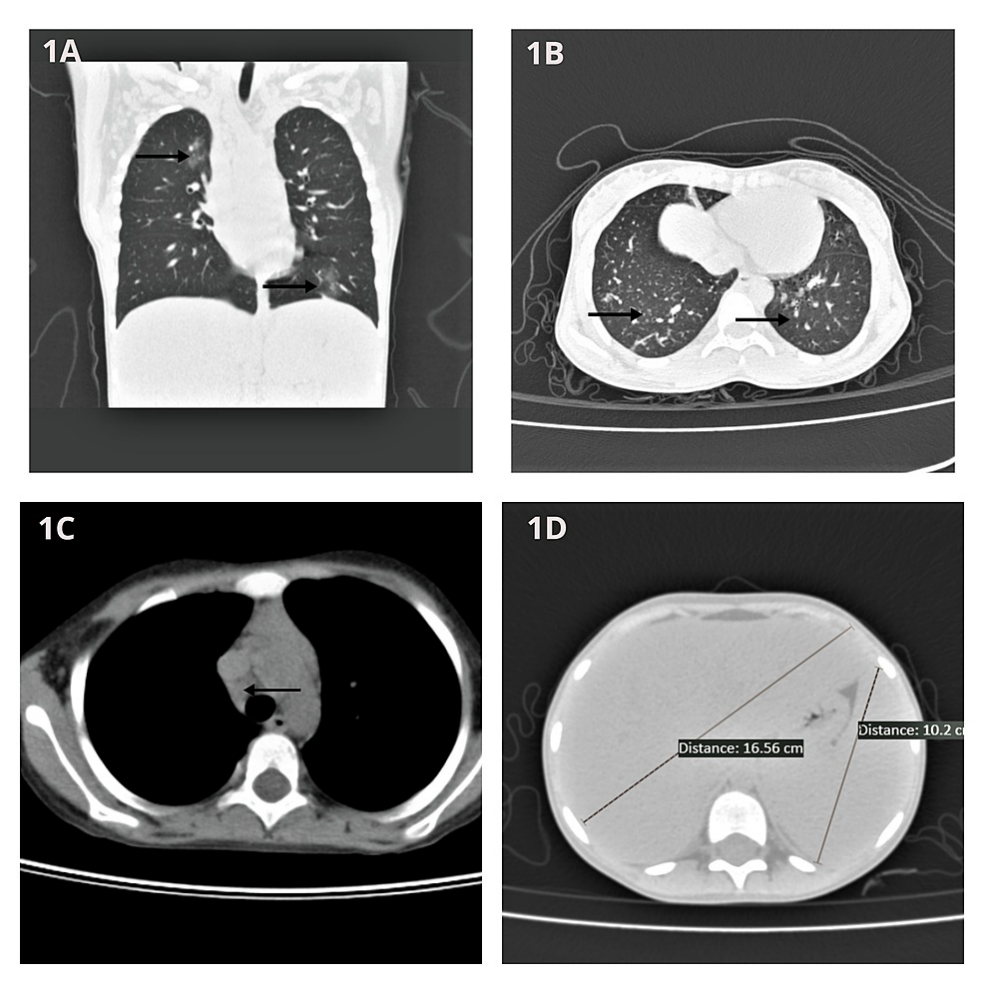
CECT of Thorax and Abdomen Bilateral areas of air-space opacifications with surrounding ground-glass opacities and interstitial thickening involving apical and anterior segments of the right upper lobe, along with antero-medial and basal segments of the left lower lobe (1A, 1B). Also noted were pretracheal and precarinal lymph nodes (1C). CECT abdomen shows hepatosplenomegaly (1D). CECT: Contrast-enhanced computed tomography
